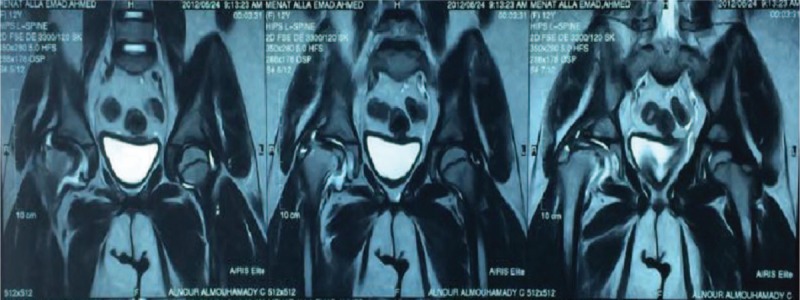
MRI of both hip joints. Note the right hip joint effusion, with deformed and collapsed right femoral head of altered signal intensity; picture is suggestive of high-grade Legg–Calvé–Perthes disease. MRI = magnetic resonance imaging.
